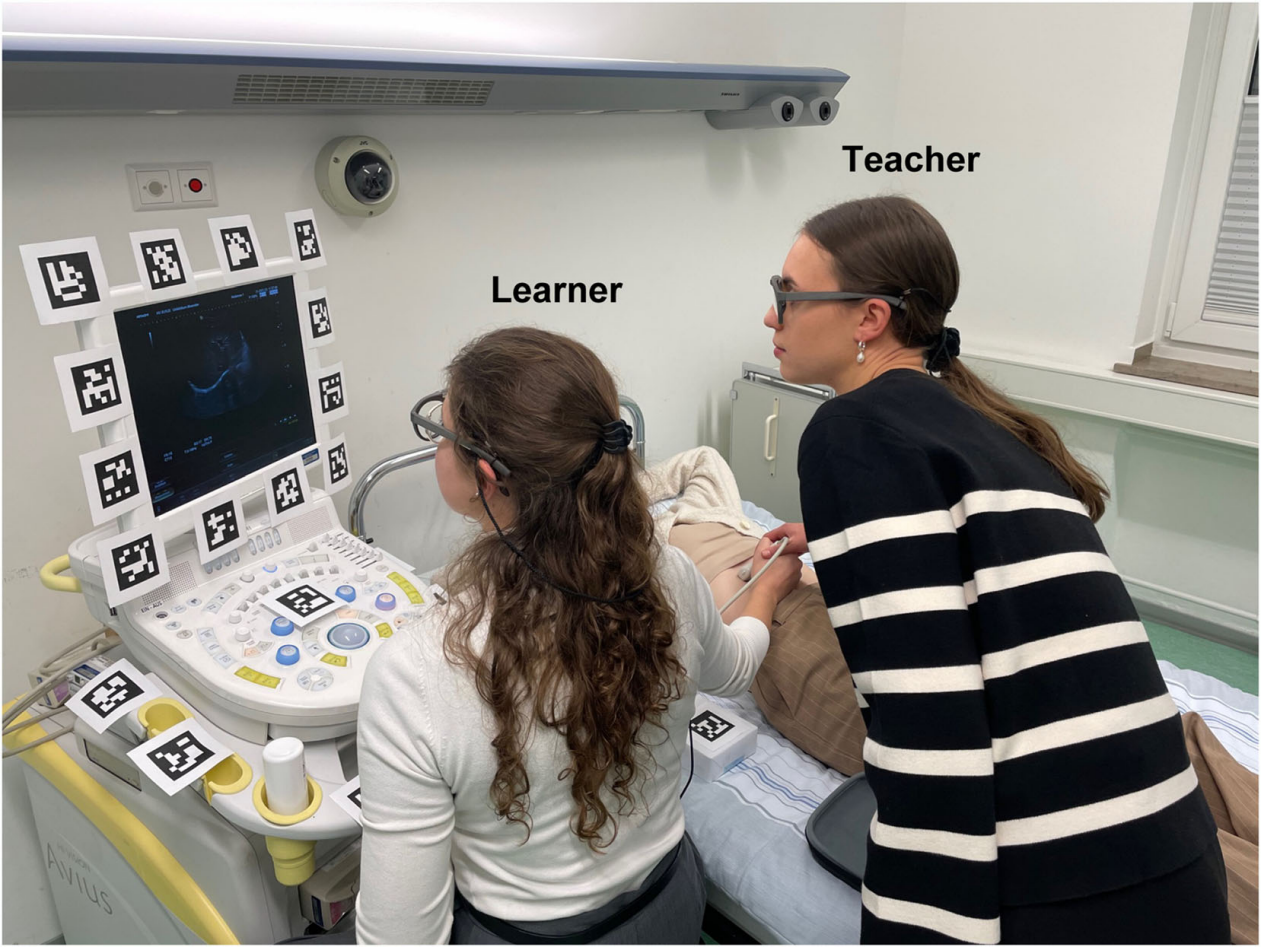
Training setup and post‐test assessments. The picture shows the training setup in a coaching scenario, where the learner is performing the ultrasound examination independently while receiving scaffolding and support from the teacher. Note that both the teacher and learner wore mobile eye‐tracking glasses to track their eye movements during the training. [Color figure can be viewed at wileyonlinelibrary.com]
